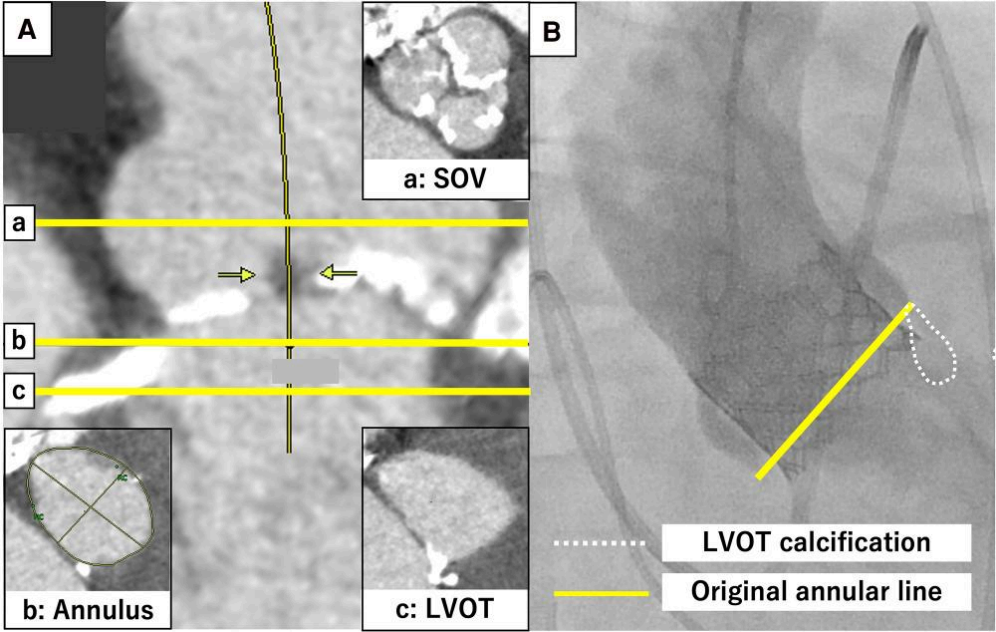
Pre- and post-procedural images of Case 3. (*A*) Pre-procedural computed tomography: a, sinus of Valsalva level; b, annular level; c, left ventricular outflow tract level. (*B*) Aortography after transcatheter aortic valve replacement.
